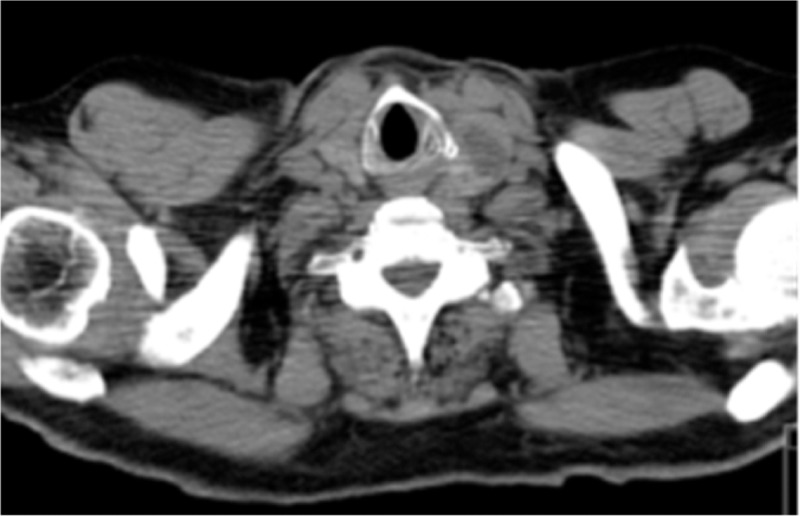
CT scan revealing diving goiter. Detailed head and neck examination is important to exclude an enlarged thyroid. Goiter was confirmed by CT scan particularly an enlarged left thyroid lobe.
